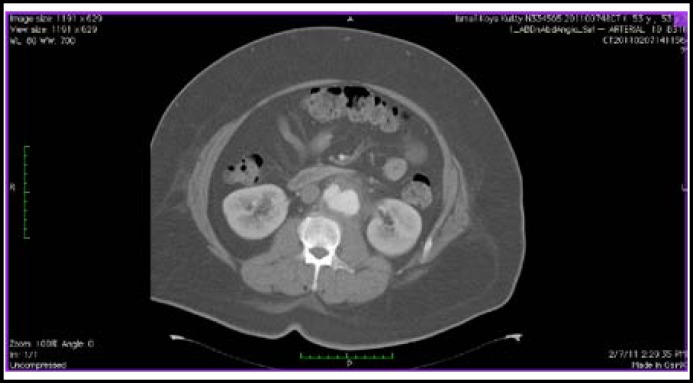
Axial CT section of the patient’s abdomen showing a left saccular infrarenal aortic aneurysm
